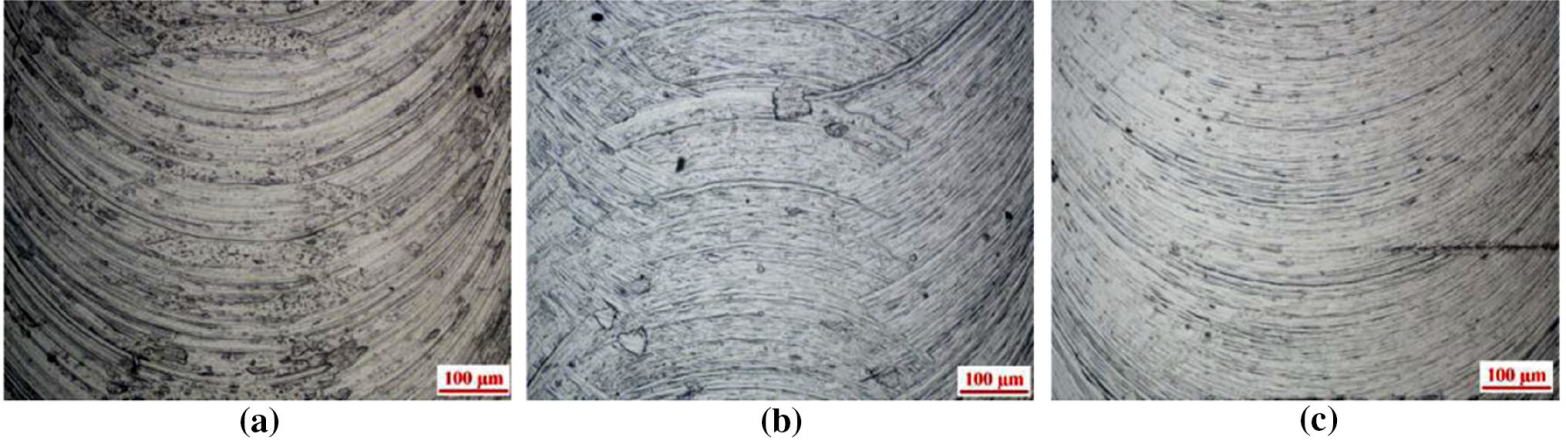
Machined surface after ultrasonic vibration micro-milling experiments at amplitudes of a 0 µm, b 2 µm, and c 4 µm, showing that ultrasonic vibration-assisted micro-milling can reduce surface defects and machining marks and thus improve the surface quality (Reprinted from “Machinablity improvement with ultrasonic vibration–assisted micro-milling” by Xu et al. [176], with permission from Sage)
